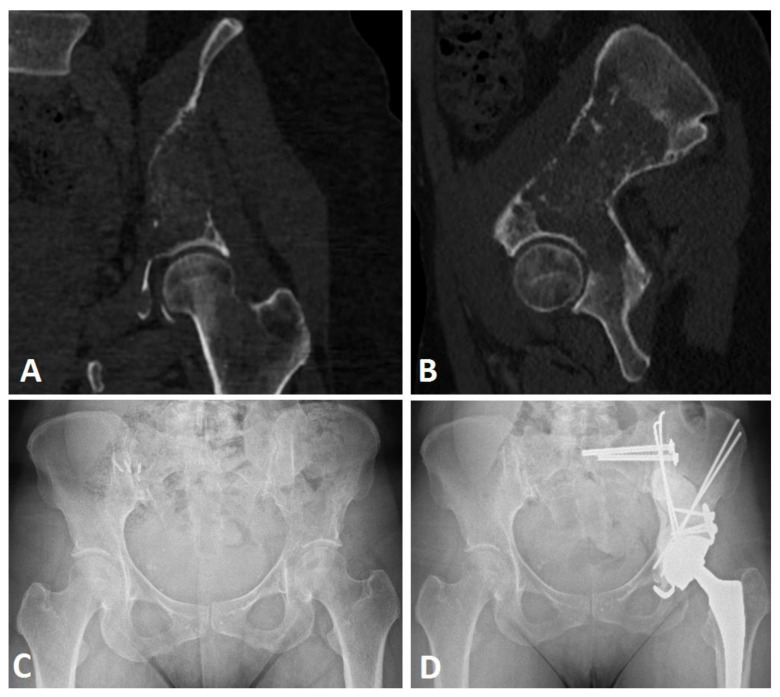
Harrington procedure (Steinman pins and cement) with THA with acetabular reinforcement using a Kerboul cross-plate and screws, and sacro-iliac joint screwing in a Harrington III lesion secondary to breast cancer. CT scan frontal (A) and sagittal (B), preoperative views, preoperative (C), and postoperative (D) AP X-ray views at 22 months of follow-up.
